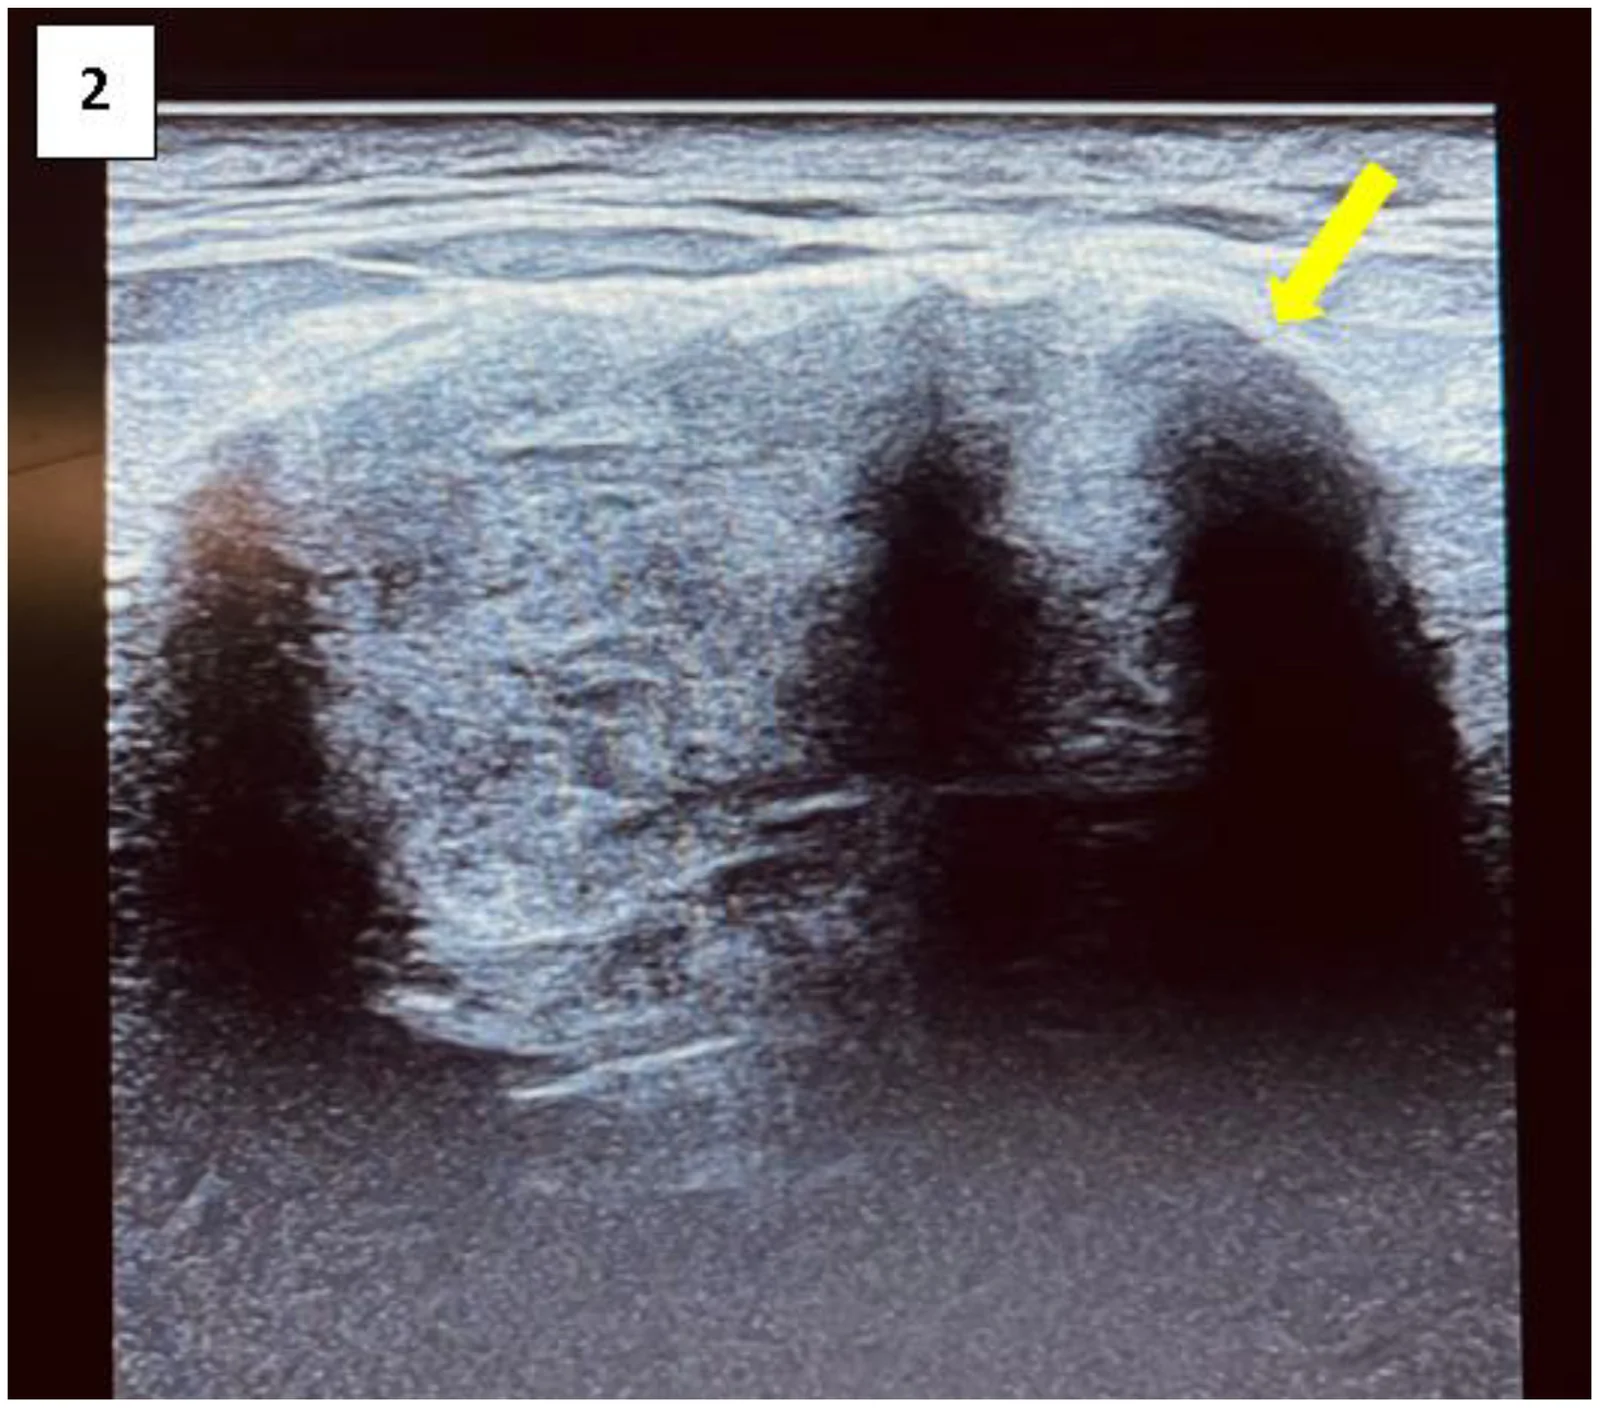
Transverse ultrasound image of the left gluteal soft tissues demonstrating a large, well-defined subcutaneous mass with lobulated margins and heterogeneous isoechoic echotexture, without internal vascularity on color Doppler. Peripheral calcifications (yellow arrow) produce posterior acoustic shadowing.
